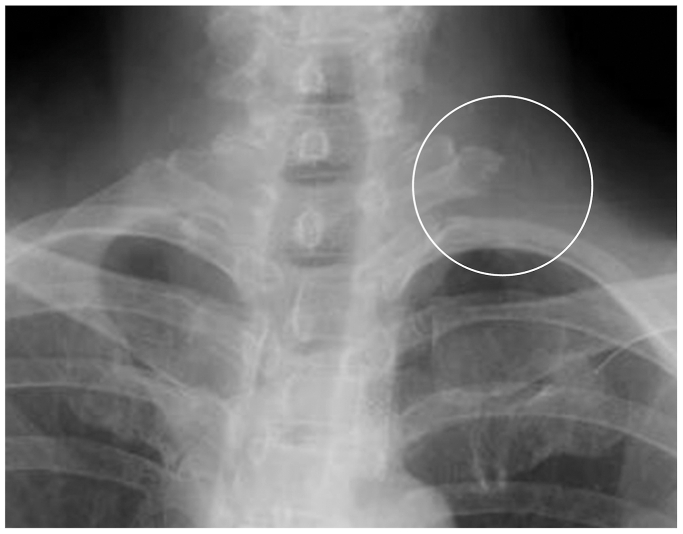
Postoperative radiographs of the first rib.
